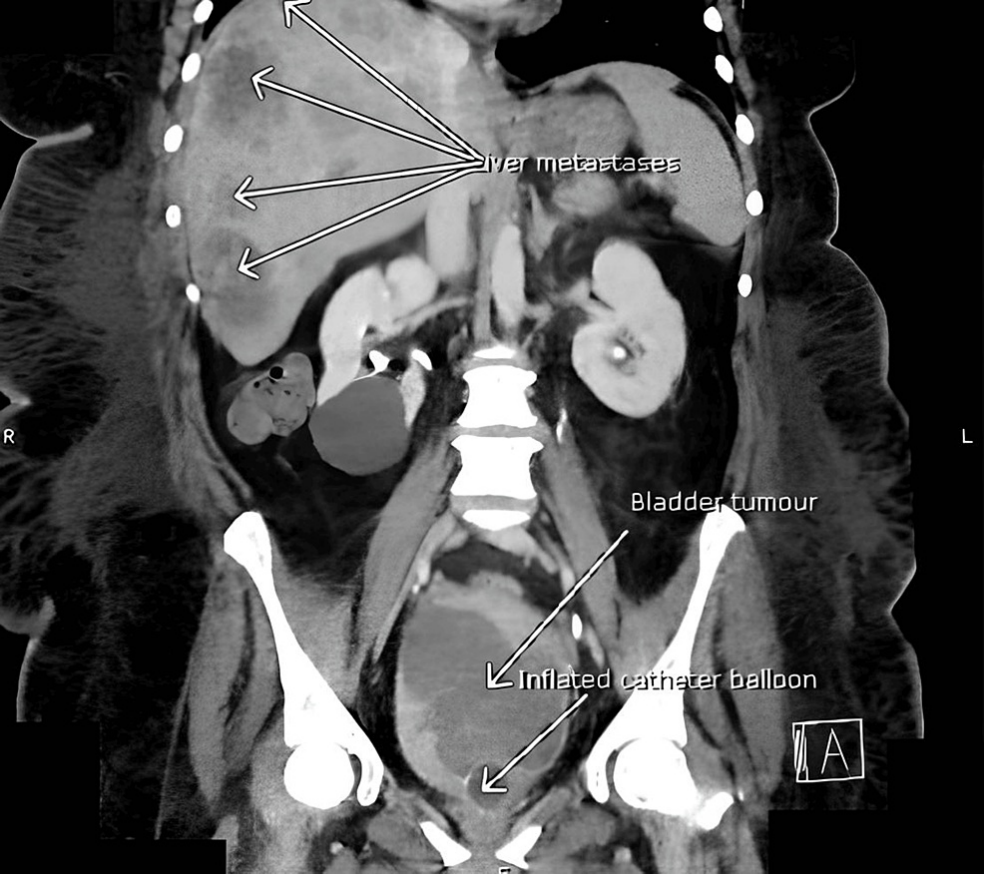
Coronal section from second CT-Urography demonstrating large bladder mass and metastatic liver disease.
